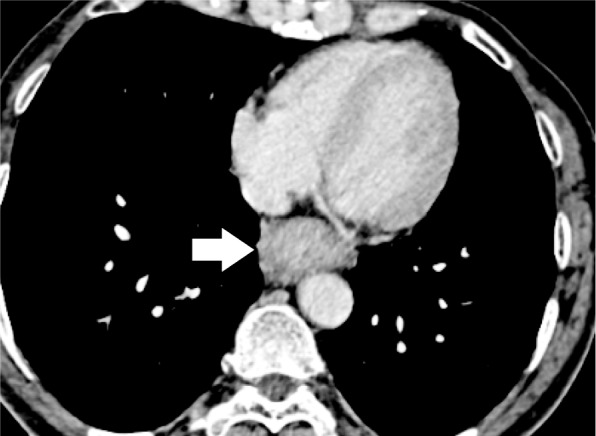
CT image before treatment. Wall thickness of the lower thoracic esophagus (white arrow)
